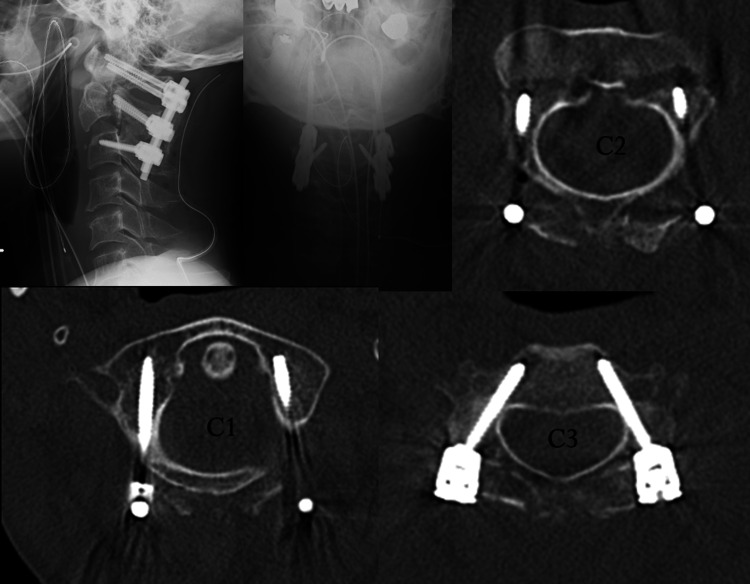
Postoperative plain X-ray and CT images No implant deviation or obvious protrusion into the vertebral artery.
